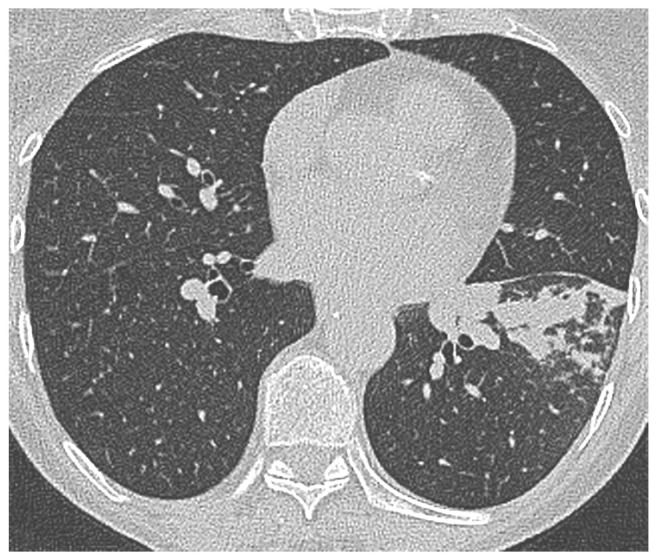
Chest CT: unilateral interstitial infiltrates with mild segmental patchy bronchopneumonic opacities and ground-glass changes, suggestive of atypical pneumonia compatible with *Chlamydophila pneumoniae*. Representative image from the anonymized institutional radiology archive of University Hospital Frankfurt.
